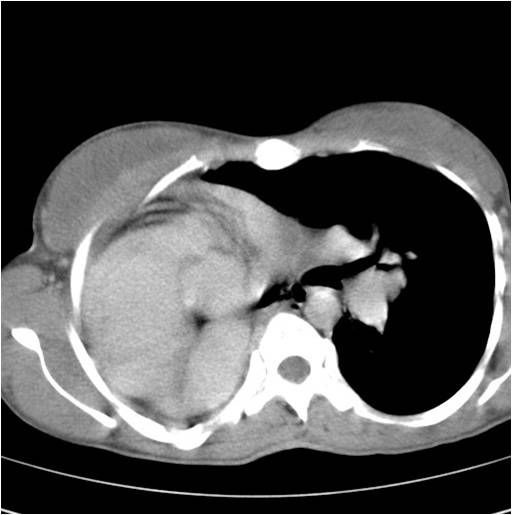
Computed tomography (CT) of the thorax, showing the total absence of the right lung with a blindly-ending right main bronchus, complete shift of the mediastinum to the right, and left lung hyperinflated with herniation to the right hemithorax.
